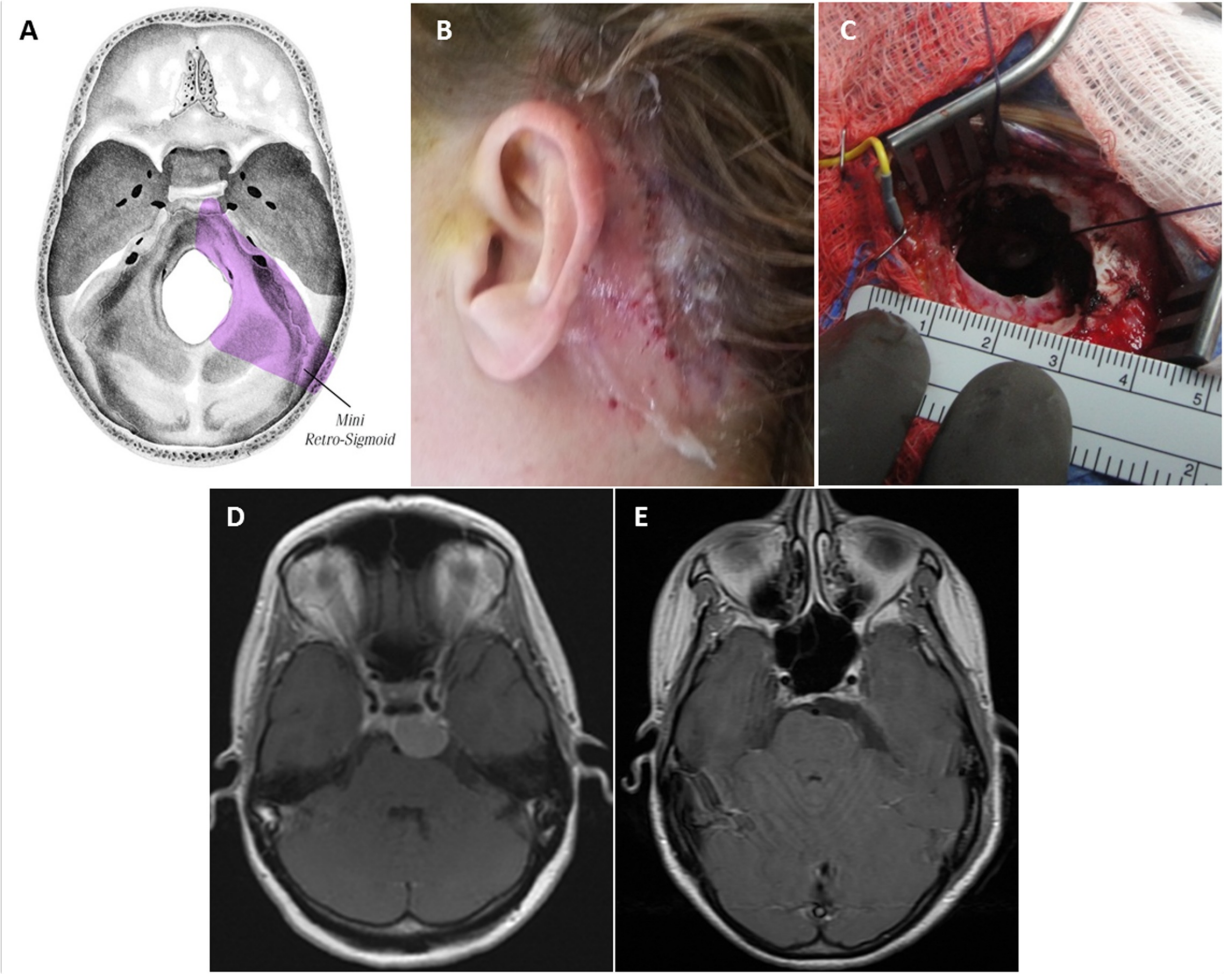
Miniature Retrosigmoid Keyhole Approach The skull base regions accessible through this approach (A). Postoperative incision site (B). Keyhole craniotomy less than 3 cm (C). Preoperative post-contrast axial T1 MRI shows complex left posterior clinoid/petroclival meningioma (D). Postoperative axial MRI (E).
